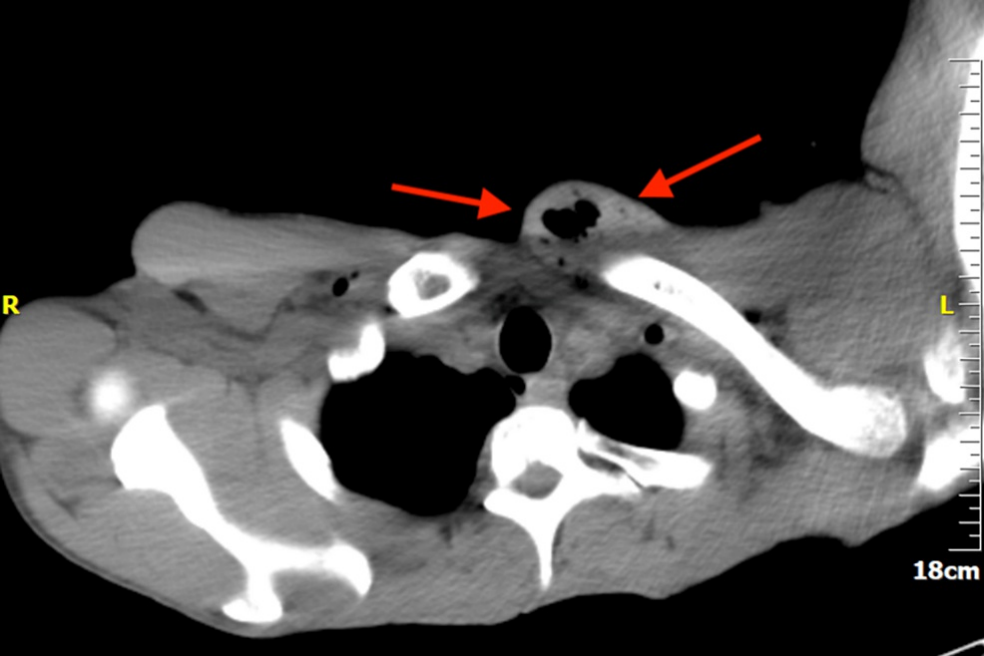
Chest CT scan, mediastinal window. CT: computed tomography
